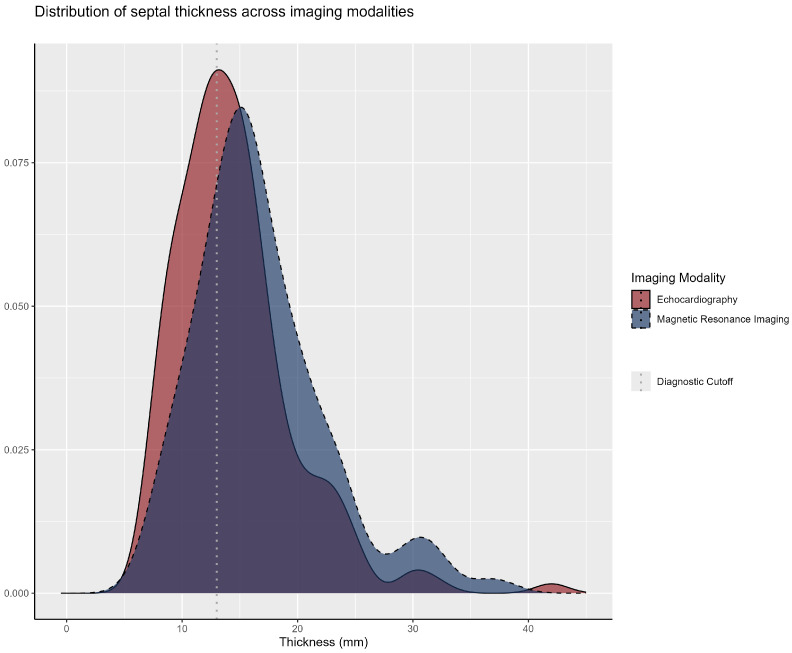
Septal thickness distribution across imaging modalities (echocardiography vs. cardiac MRI). The distribution of interventricular septal thickness (measured in millimeters) according to imaging technique: echocardiography (solid red line) and cardiac MRI (dashed bluenline). The vertical line at 13 mm (dark gray dotted line) represents the diagnostic cutoff for hypertrophic cardiomyopathy (HCM) as defined by the 2024 AHA/ACC/AMSSM/HRS/PACES/SCMR guideline.
